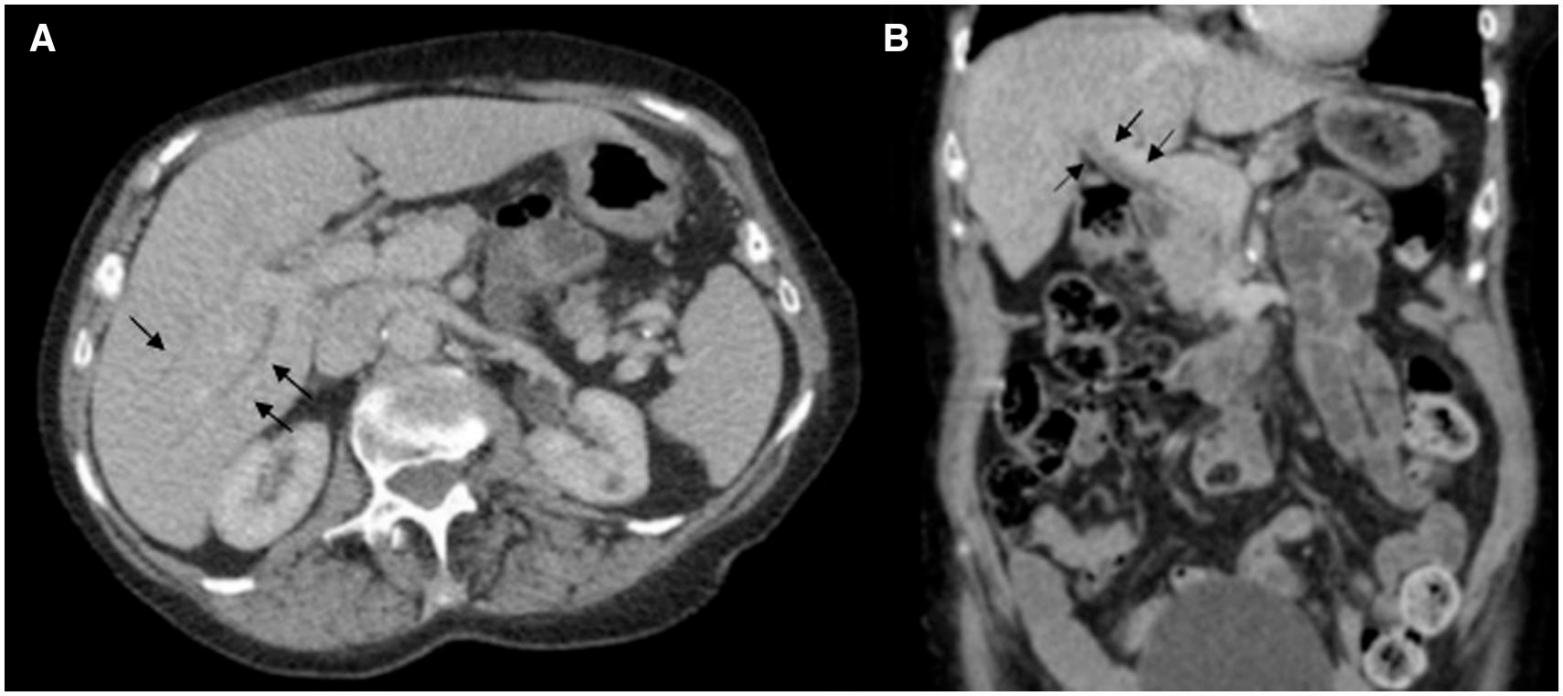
CT performed in February 2021: (A) Axial contrast-enhanced CT image showing mild intrahepatic biliary dilatation with enhancing diffuse ductal wall thickening, but less thickened than in previous study in Figure 1, concerning for cholangitis (black arrows). (B) Coronal reformatted image showing mildly dilated common hepatic and common bile duct with enhancing thickened wall (black arrows).
